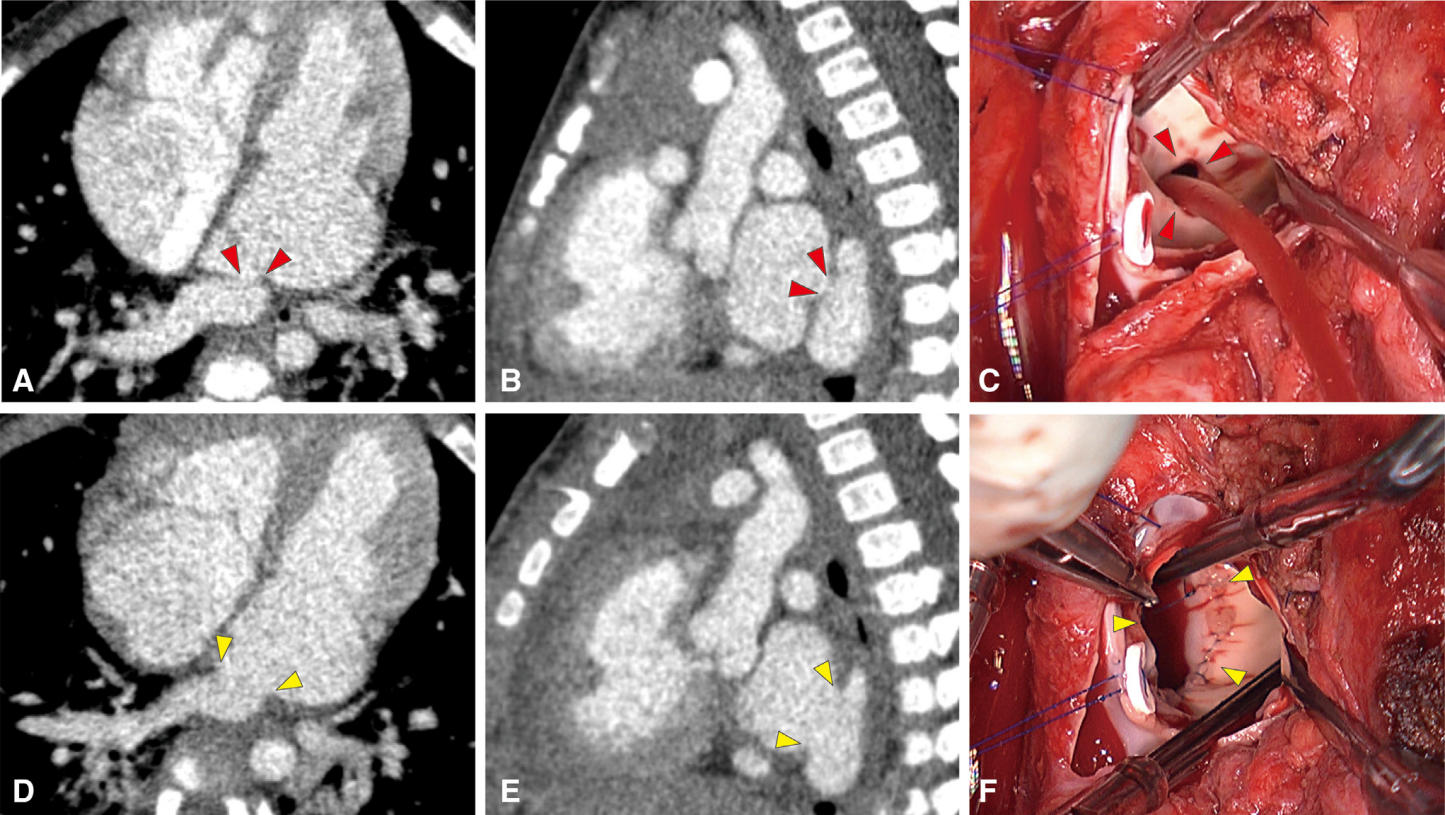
Pre- and postrepair images. A and B, Pre-repair computed tomography (*CT*) images showing the stenotic orifice (*red arrowheads*). C, Intraoperative image showing the stenotic orifice through the incised interatrial septum (*red arrowheads*). D and E, Postrepair CT images showed a widely opened orifice (*yellow arrowheads*). F, Intraoperative picture showing the enlarged orifice (*yellow arrowheads*).
